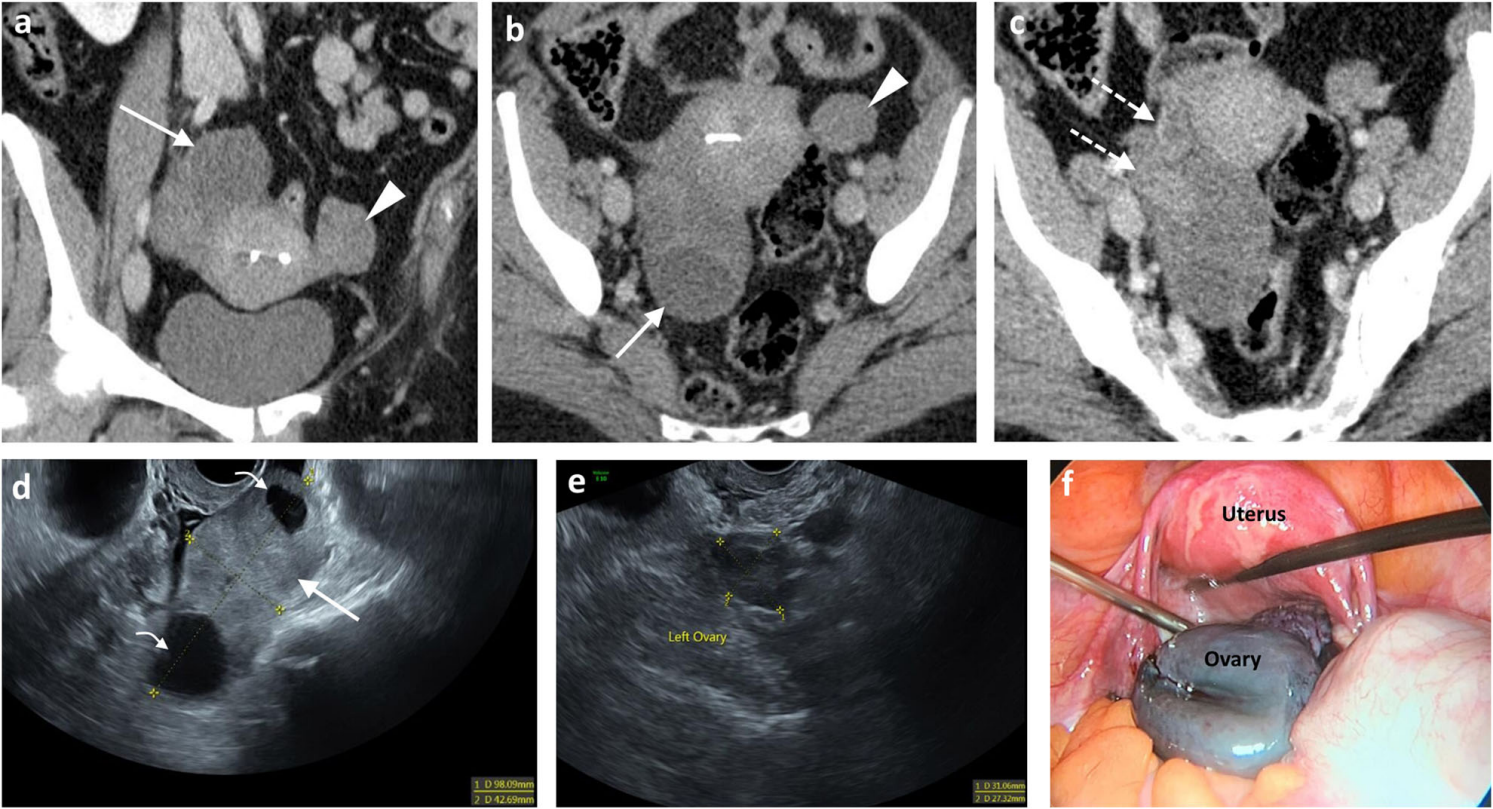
Right adnexal torsion in a woman in her 40’s with intense right pelvic pain and vomiting. CT was requested to exclude appendicitis. a–c Post-contrast coronal (a) and axial CT images (b, c) excluded appendicitis and showed an enlarged and hypodense right ovary (arrow) posteriorly displaced behind the uterus in the axial images, compared to the normal size and located left ovary (arrowhead) and twisted right fallopian tube (dotted arrows). d, e Subsequent transvaginal US performed preoperatively by the gynaecologist confirmed the CT findings with an enlarged and oedematous right ovary (arrow) measuring approximately 98 × 42 mm with distended peripheral follicles (curved arrows). f Intraoperative image confirmed right ovarian necrosis
